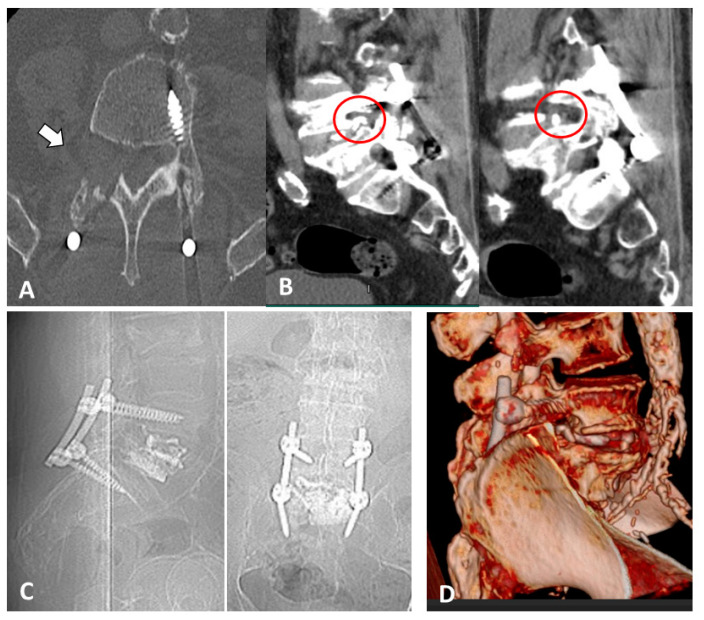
The 3-month follow-up, post-operative imaging. (A) Axial CT scan at the L4–L5 right neuroforamen, showing the foramen enlargement after hemilaminectomy and foraminotomy (white arrow); (B) sagittal CT scans showing neuroforamen enlargement over cement leakage (red circle); (C) sagittal (left) and coronal (right) post-operative X-rays; (D) 3D CT reconstruction of the lumbar spine: foramen enlargement over cement leakage.
